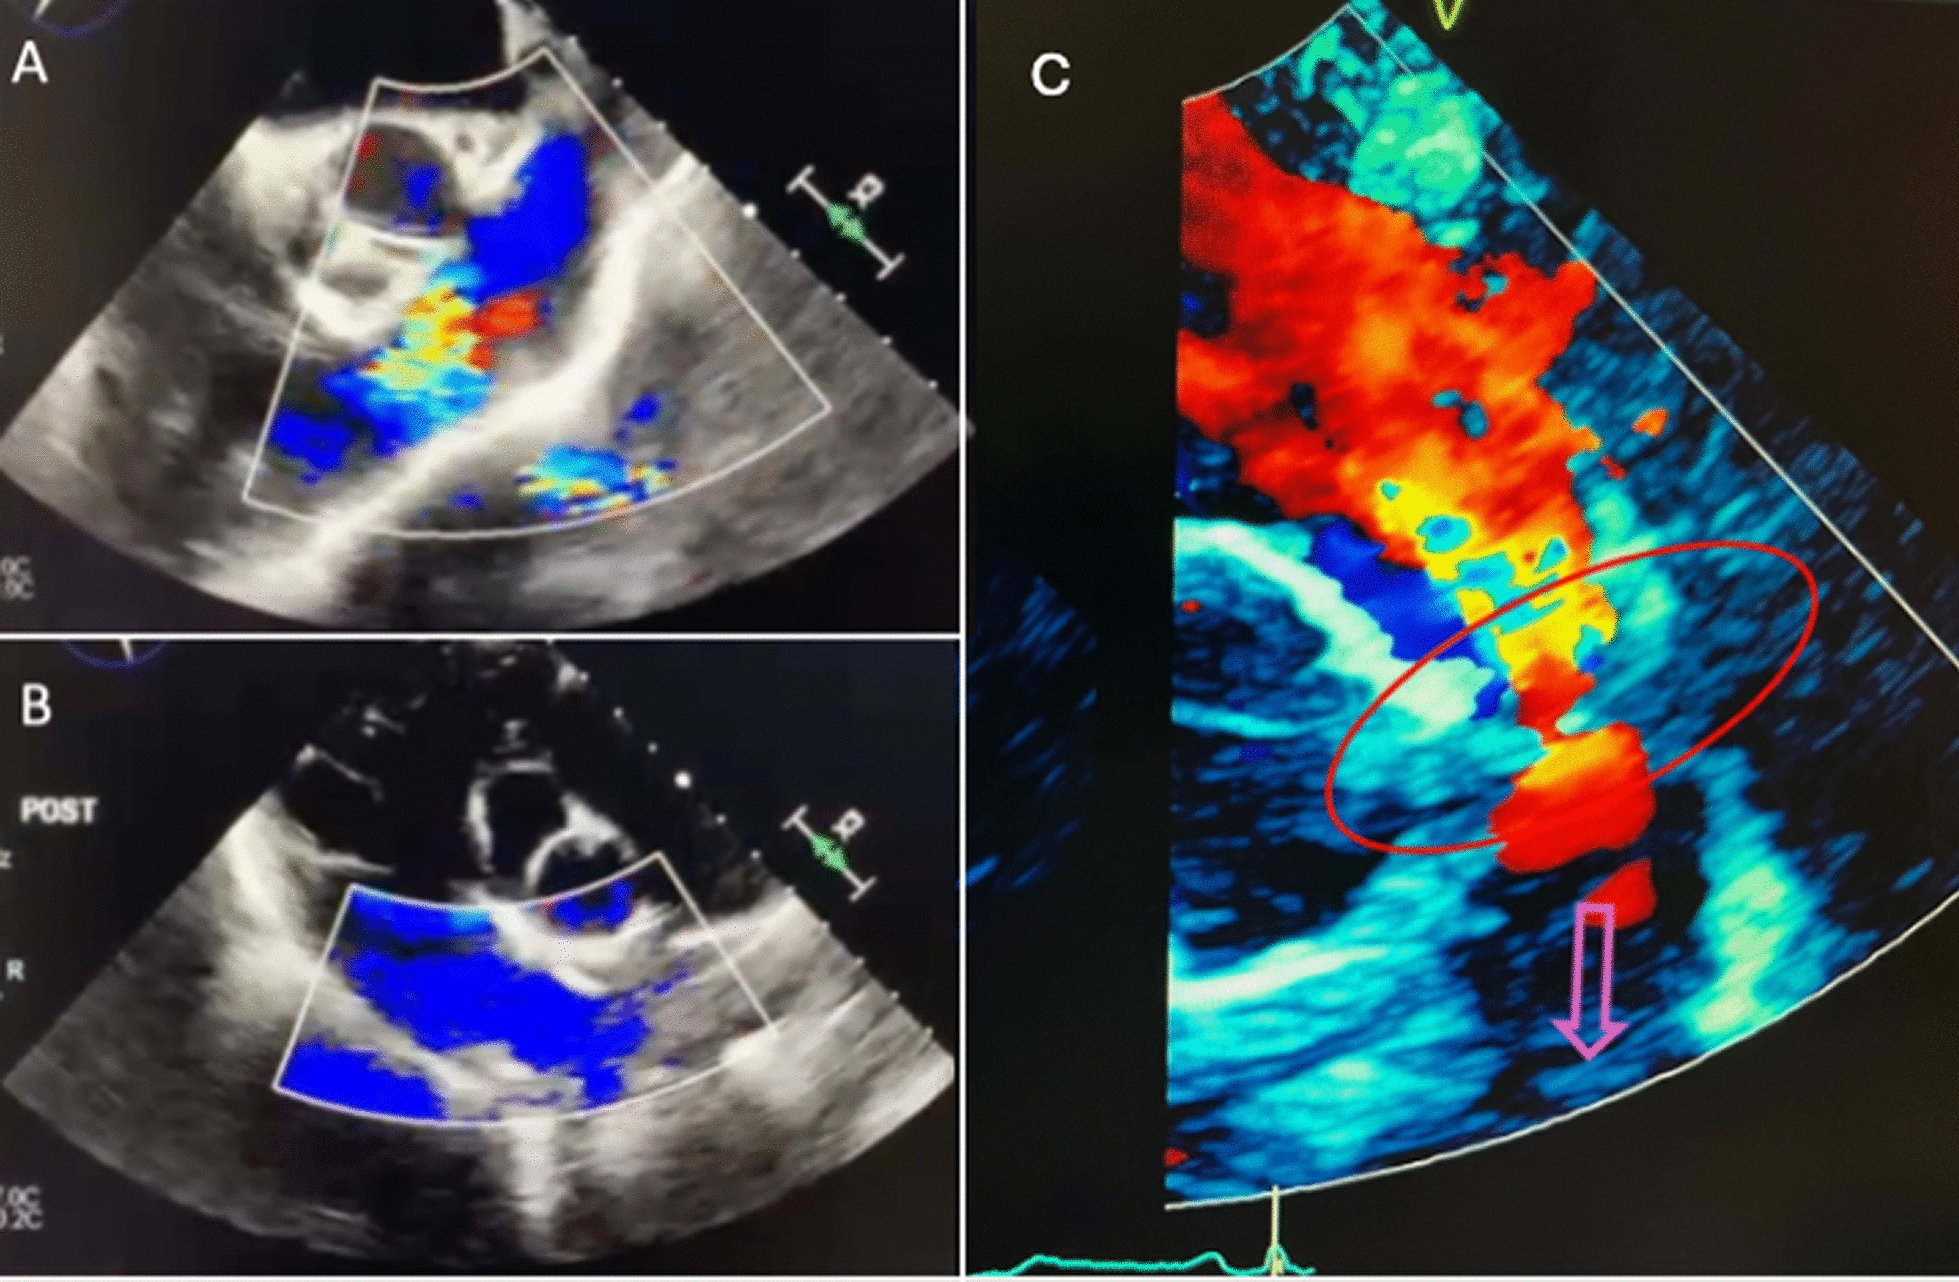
Intraoperative transesophageal echocardiogram of right ventricular inflow outflow view before A and after B pulmonary valve replacement showing severe pulmonary regurgitation before cardiopulmonary bypass and competent IR bioprosthetic valve after replacement. C Transthoracic echocardiography 17 months after implantation demonstrating moderate-severe regurgitation. Red oval: pulmonary valve; Purple arrow: pulmonary artery
